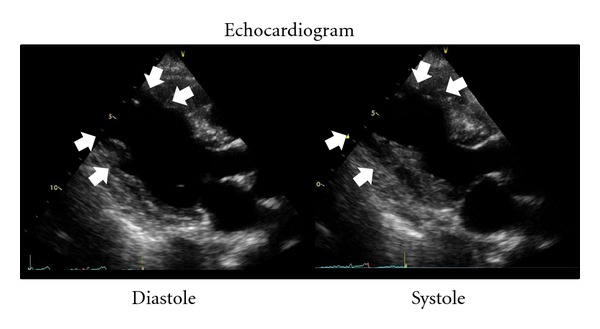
These images show echocardiography obtained at circulatory failure (41 hours after taking Basta) through cardiac cycle. There is akinesis lesion from mid to apex of left ventricle (LV) through cardiac cycle (white arrow). Meanwhile, there is hyperkinetic motion at base of LV.
